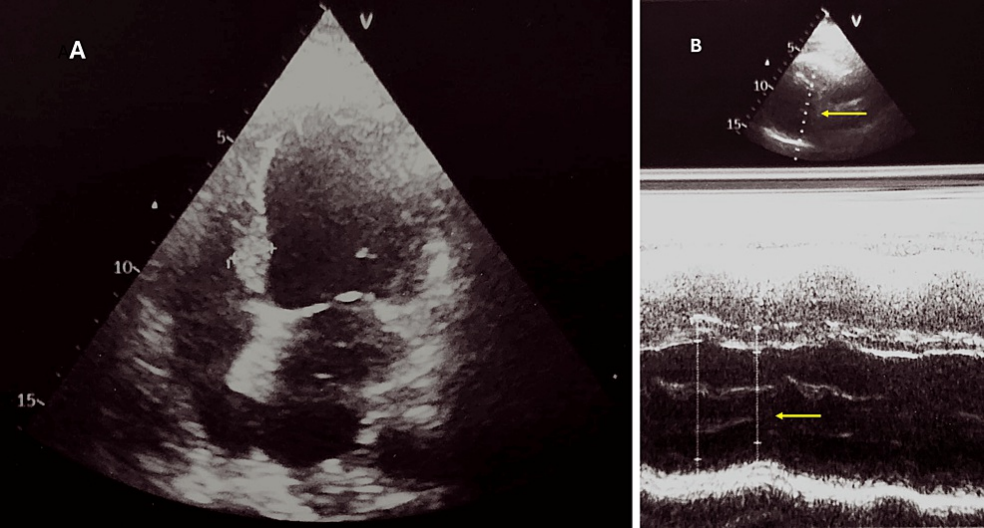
Transthoracic echocardiography (A) Apical four-chamber echo view, and (B) parasternal long axis view showing measurements (arrows) of interventricular septal diameter, end-diastolic left ventricular diameter, end-systolic ventricular diameter, and left ventricular posterior wall diameter
